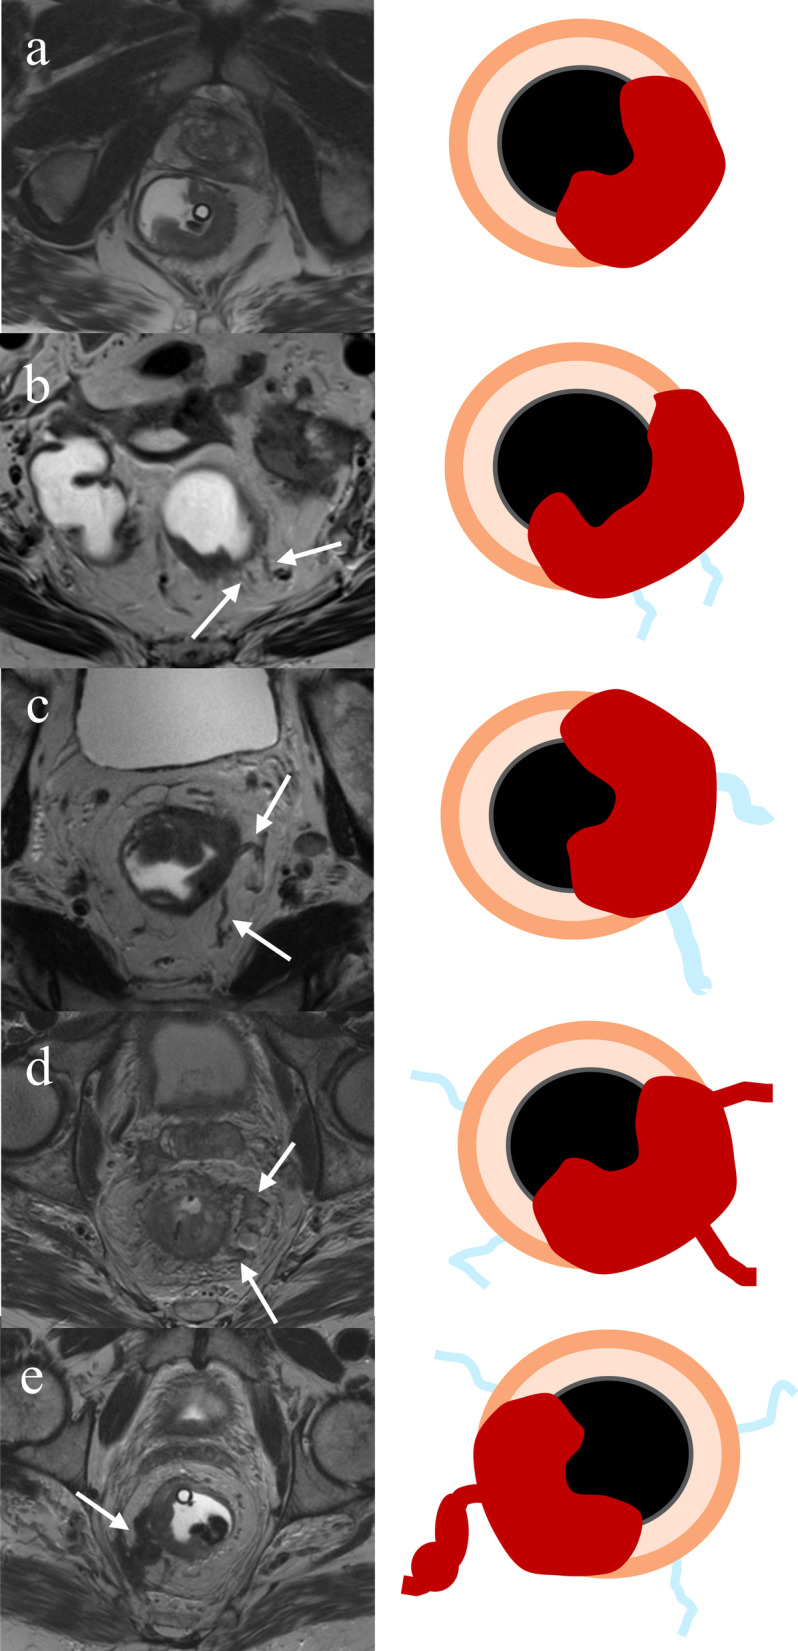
Extramural venous invasion scoring system based on T2-weighted images. a No vessel exists adjacent to the primary tumor (score 0). b Normal diameter vessel adjacent to the primary tumor demonstrates no tumor signal intensity (score 1). c Slightly expanded vessel without abnormal signal intensity (score 2). d Expanded vessel including obvious tumor signal intensity (score 3). e Expanded vessel with irregular or nodular contour containing tumor signal intensity (score 4). Adapted from Smith NJ, et al. Br J Surg 2008 [22]
